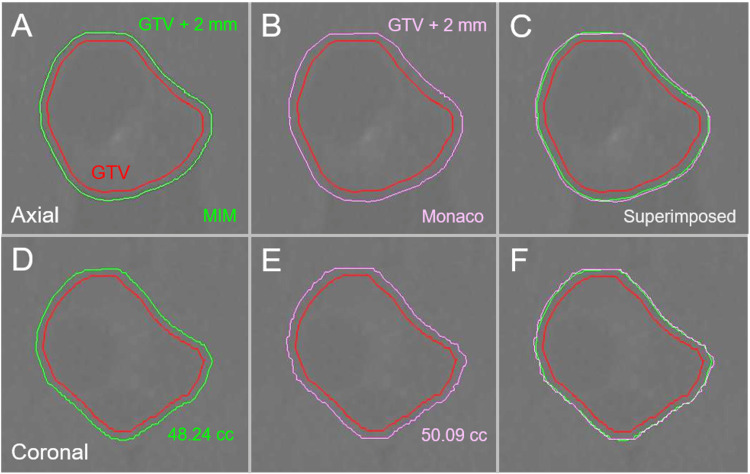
Differences in margin addition functions between two systems in clinical brain metastasis. The images show target contours superimposed onto non-CE-CT images (A-F); axial images (A-C); coronal images (D-F); by MIM Maestro (A, D); by Monaco (B, E); and the superimposed images (C, F). Isotropic 2-mm margins were added to the identical GTV (35.74 cc) of clinical BM by the two systems. The 2-mm margin-added volume by Monaco is slightly larger than that by MIM Maestro. GTV: gross tumor volume; GTV + 2 mm: GTV evenly expanded by 2 mm; MIM: MIM Maestro; CE: contrast-enhanced; CT: computed tomography; BM: brain metastasis.
